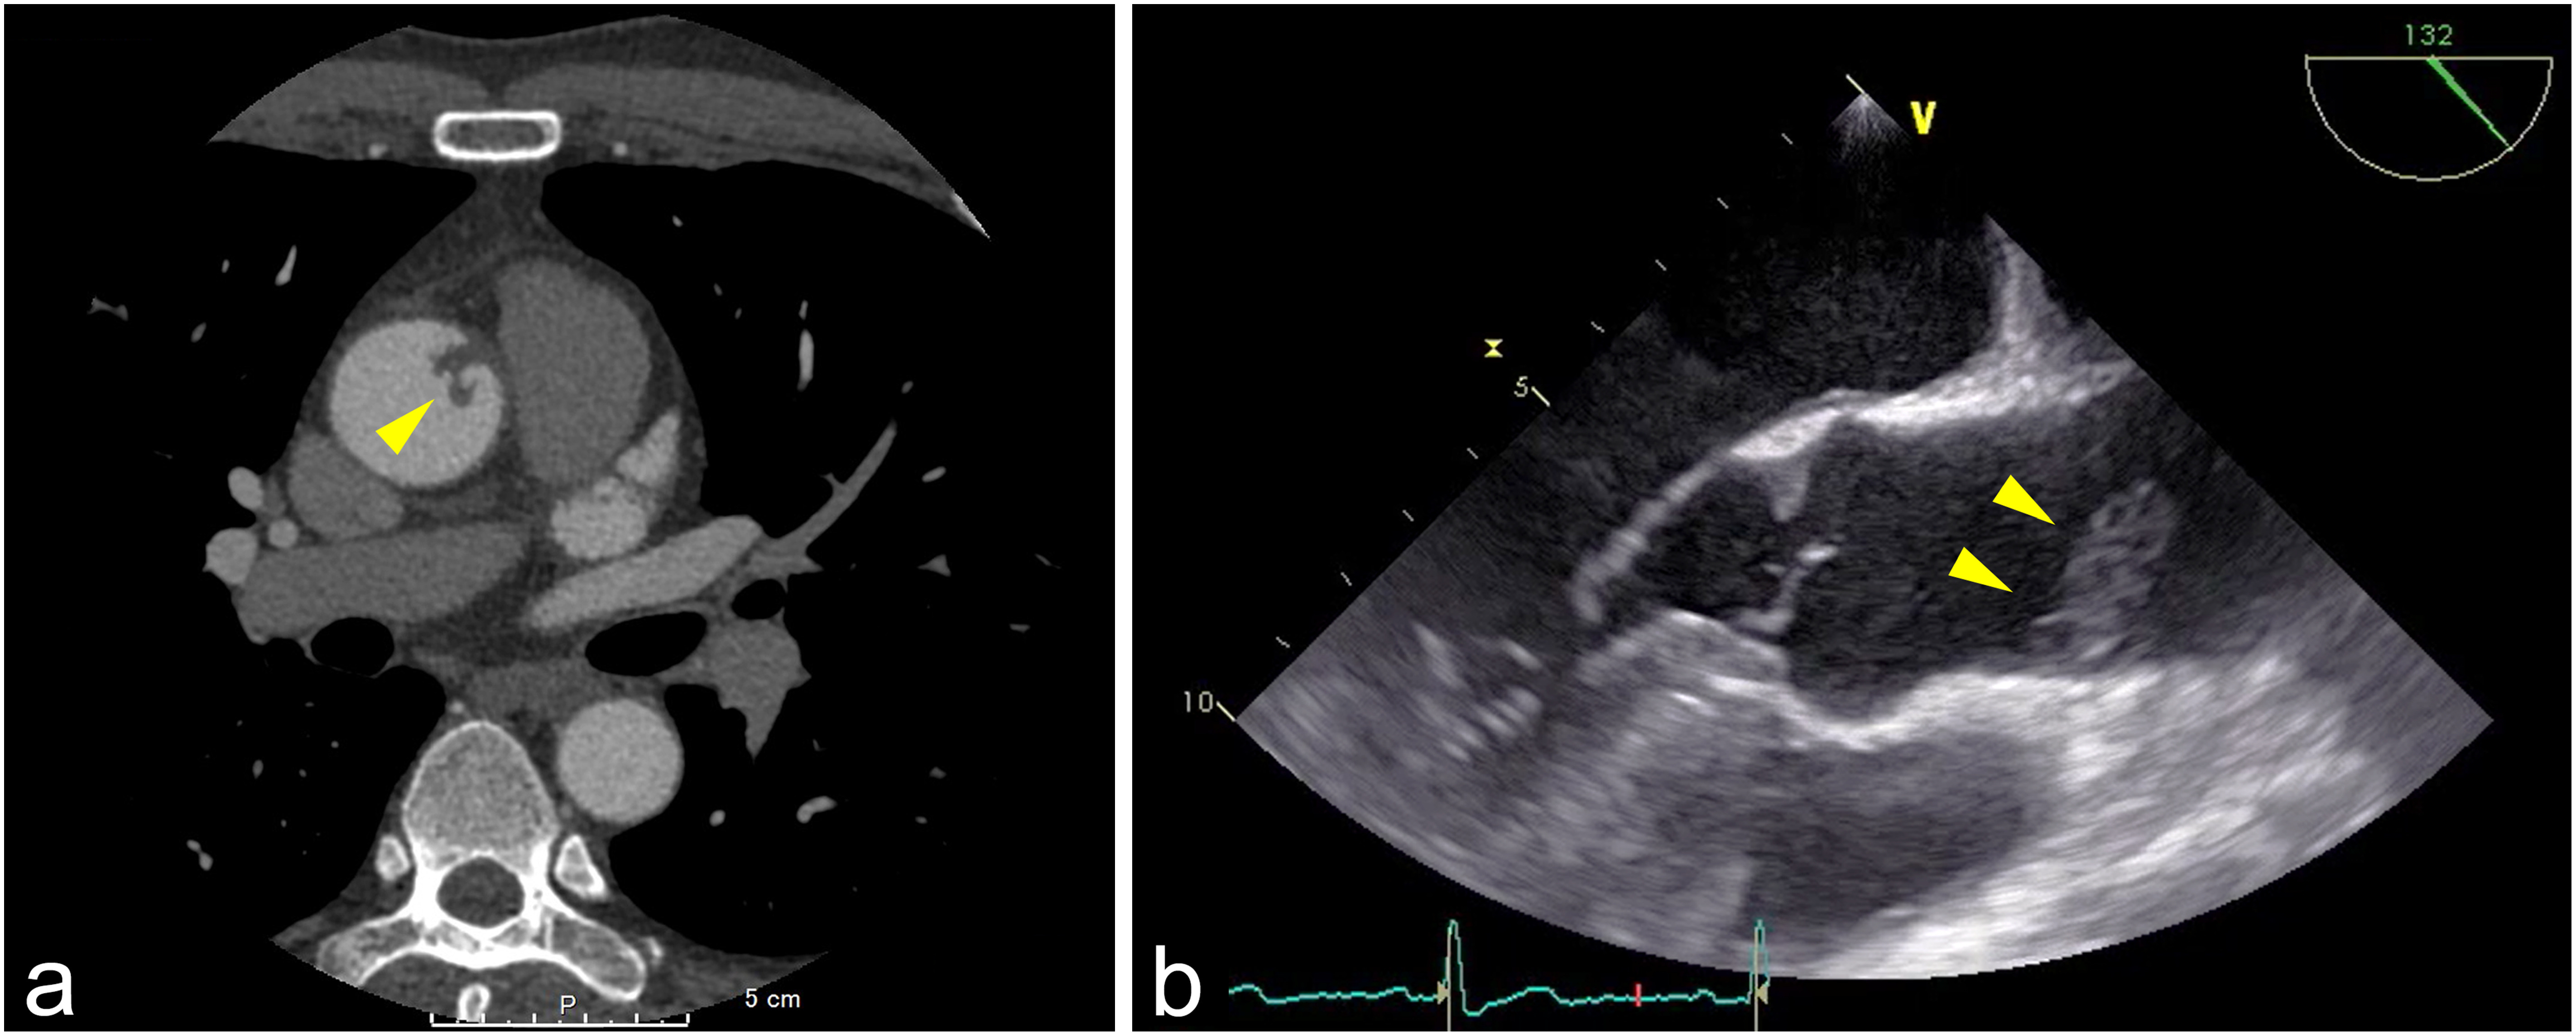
Fig. 1 (a) Computed tomography scan and (b) transesophageal echocardiogram showing a mass (arrowheads).
